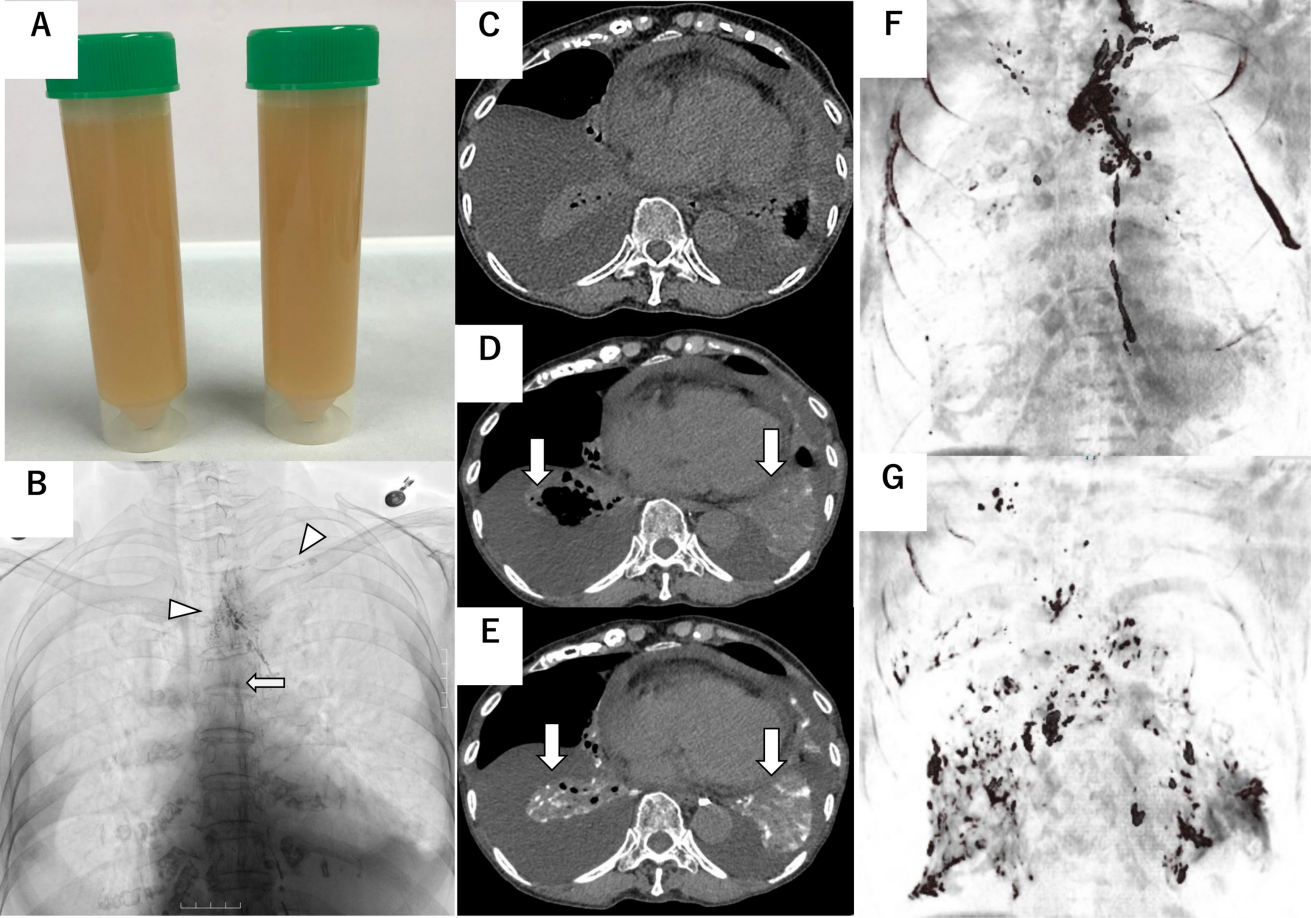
Lymphangiographic and computed tomography (CT) findings with image isolating the contrast‐enhanced structures. (A) Cloudy yellow pleural fluid obtained via thoracentesis. (B) Lymphangiography revealed a thoracic duct obstructed at the level of the carina, cranial to the lung hilum (arrow), without evidence of direct thoracic duct leakage. The contrast medium appears to reflux into lymphatic vessels surrounding the aorta and left subclavian artery (arrowheads). (C) Pre‐lymphangiography CT showing no abnormal high‐attenuation foci. (D) Immediate post‐contrast CT (without intravenous contrast) showing deposition of contrast in the lungs (arrows). (E) Follow‐up CT performed the next day (without intravenous contrast) showing more extensive and intensified bilateral contrast accumulation in the lung parenchyma, particularly near the visceral pleura, compared with the immediate post‐contrast CT (arrows). (F) Contrast‐enhanced structures extracted from the CT shown in (D), highlighting the thoracic duct and mediastinal lymphatic vessels. (G) Contrast‐enhanced structures extracted from the CT shown in (E), demonstrating enhancement of peripheral lymphatic vessels in the perihilar and subpleural regions, whereas the thoracic duct was not visible.
